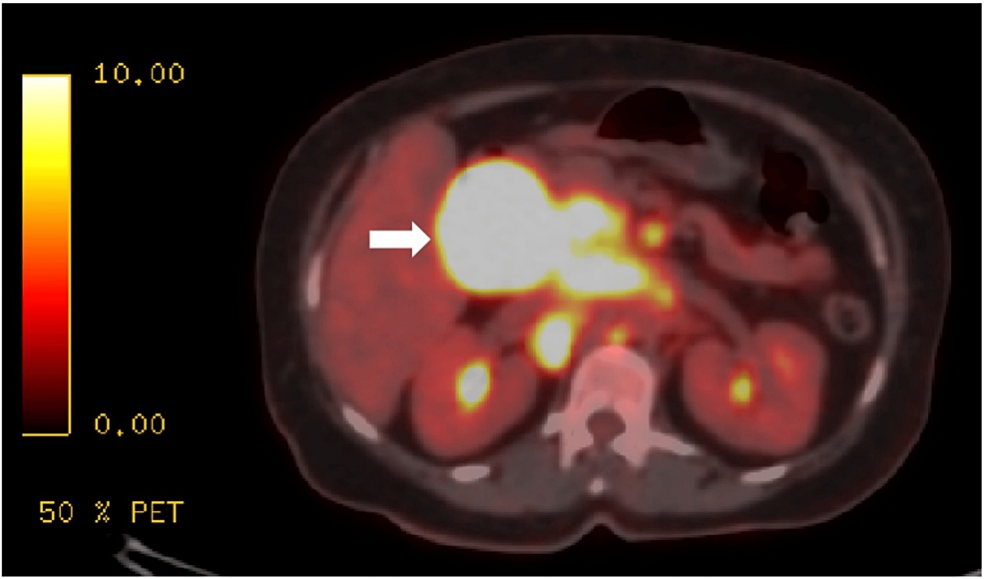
Initial positron emission tomography/CT revealed conglomerated para-aortic and gastrohepatic lymph nodes (the largest porta-hepatis lymph node is visually indicated by a white arrow).
